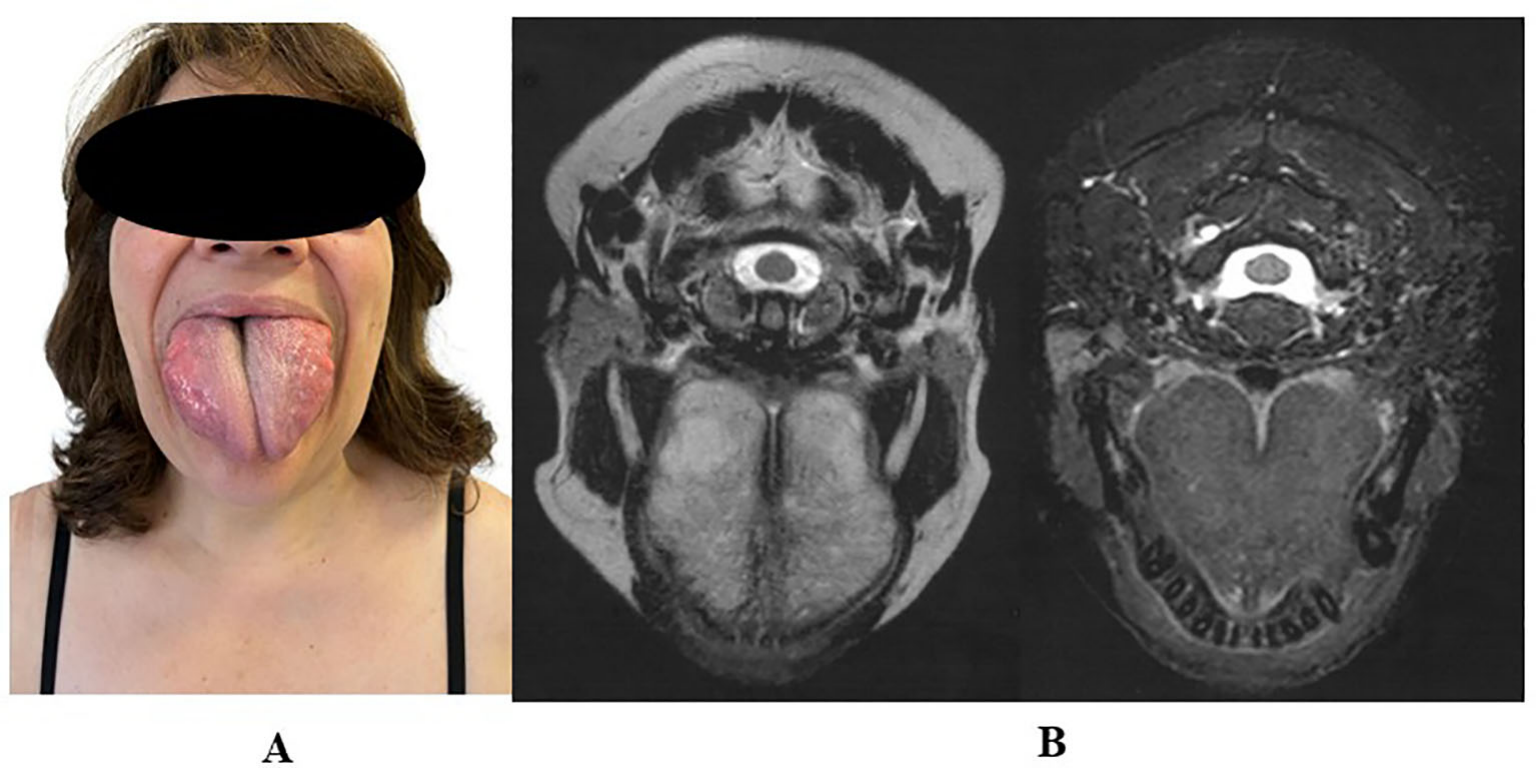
(A) Clinical image showing macroglossia in patient 5. (B) Tongue MRI findings in the same patient, diagnosed with familial partial lipodystrophy type 6 (FPLD6) and carrying a homozygous *LIPE* variant. The MRI demonstrates diffuse enlargement of the tongue, consistent with macroglossia.
